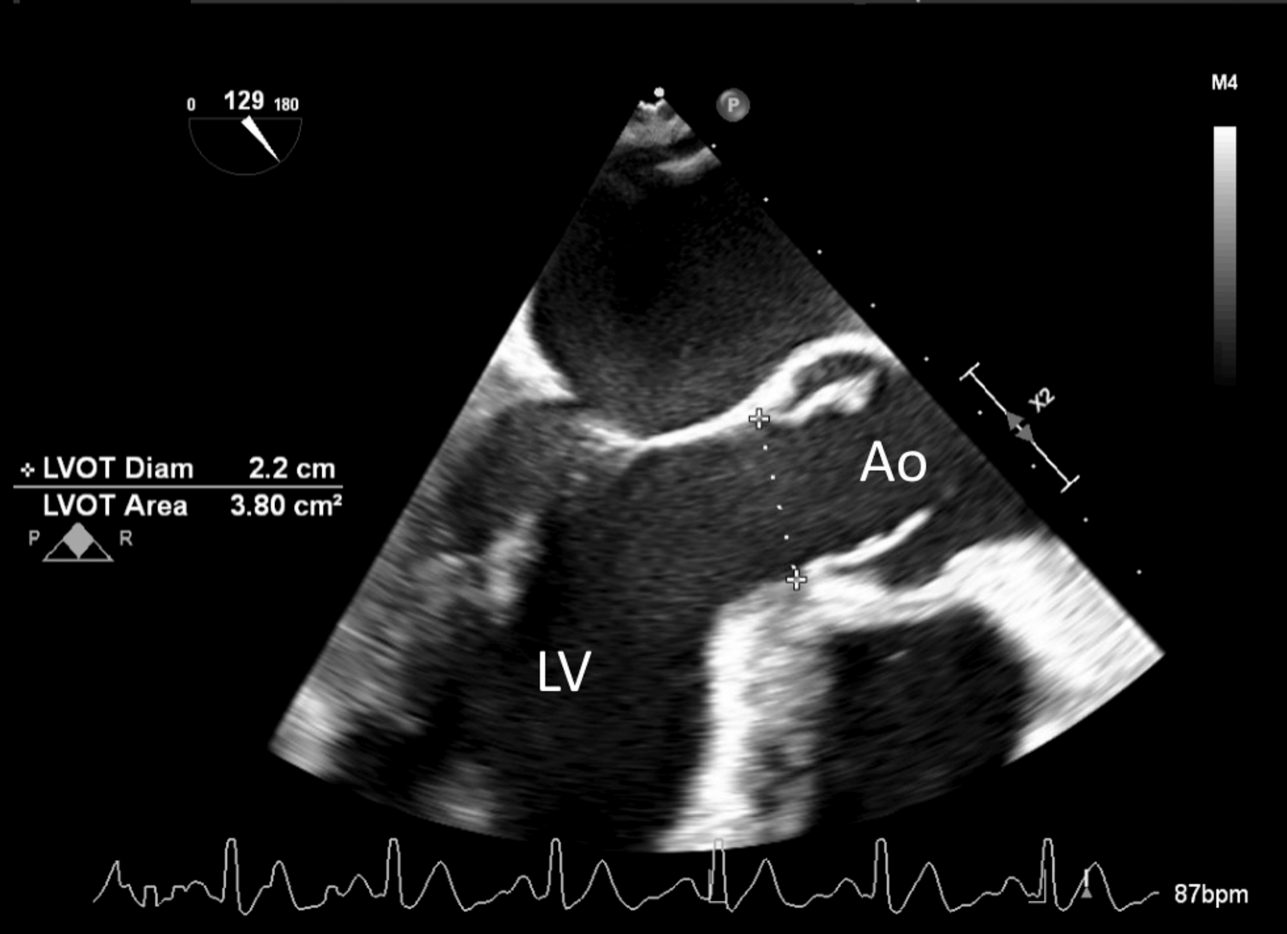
Transesophageal echocardiography (TEE) images showing left ventricular outflow tract without vegetations Ao: Aorta; LV: Left ventricle; LVOT: Left ventricular outflow tract.
